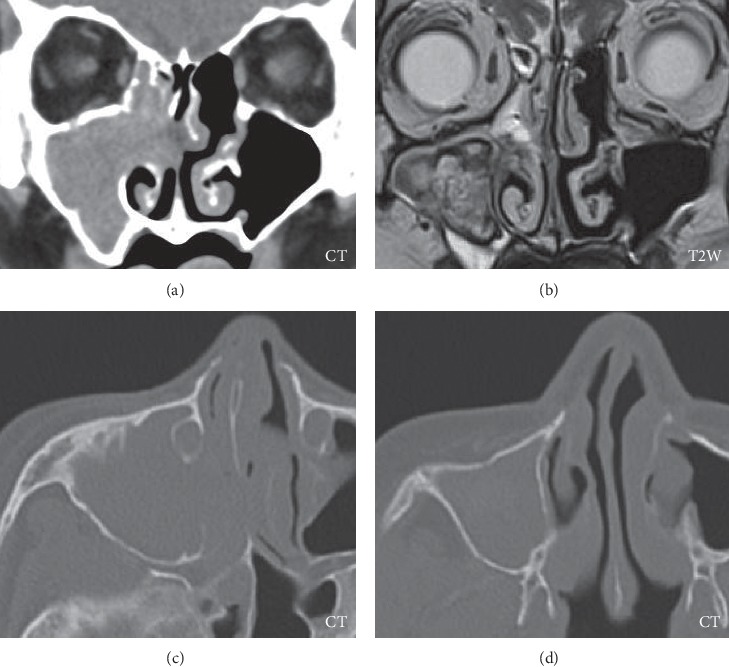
Initial images. CT: shadow of the soft-tissue density of the right maxillary sinus; T2W: well-circumscribed, smoothly marginated tumor with a peripheral low area and a central inhomogeneous area.
